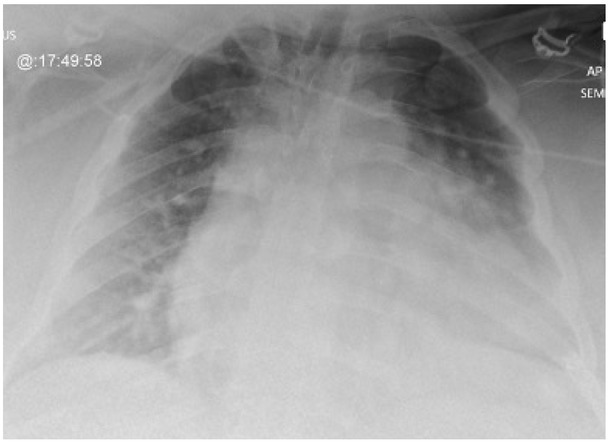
Chest X-ray of Case 2: Hazy opacification of both lungs’ mid and lower zones. Infection versus congestion.
